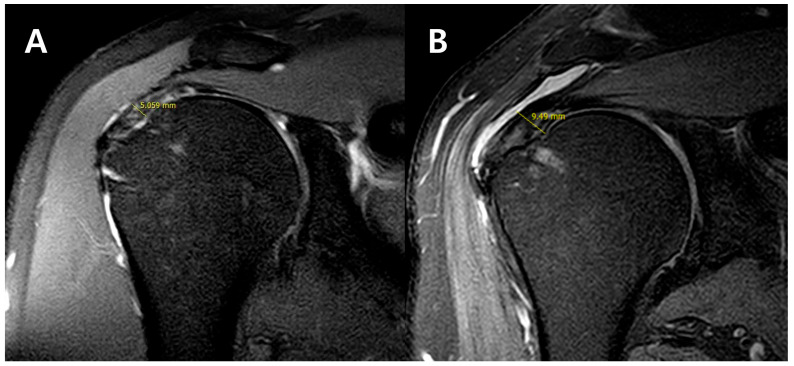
T2-weighted magnetic resonance imaging (MRI) of a 57-year-old male patient who underwent rotator cuff repair at another institution 3 years earlier: (A) Preoperative MRI demonstrating more than a 25% reduction in supraspinatus tendon thickness compared with the contralateral side, with a measured thickness of 5.059 mm. (B) Postoperative MRI obtained 1 year after surgery showing an increased supraspinatus tendon thickness of 9.49 mm.
